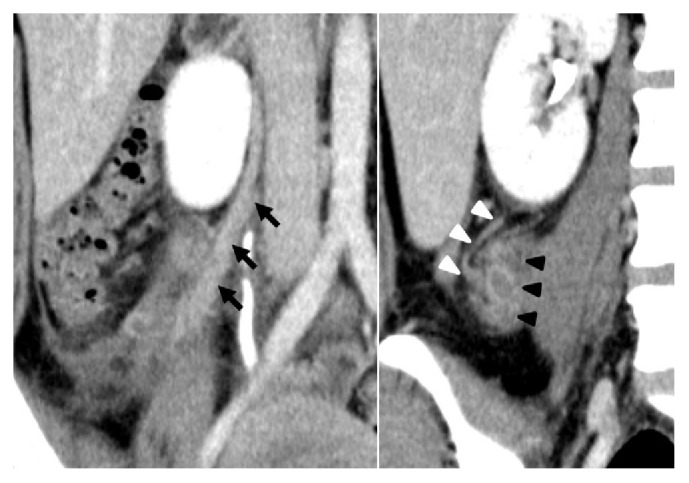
Abdominal dynamic CT scan reconstructed after surgery (delayed phase). Intact right ovarian vein (black arrow) (left). The retroperitoneal mass (black arrowhead) was connected to the right renal capsular vein (white arrowhead) (right).
